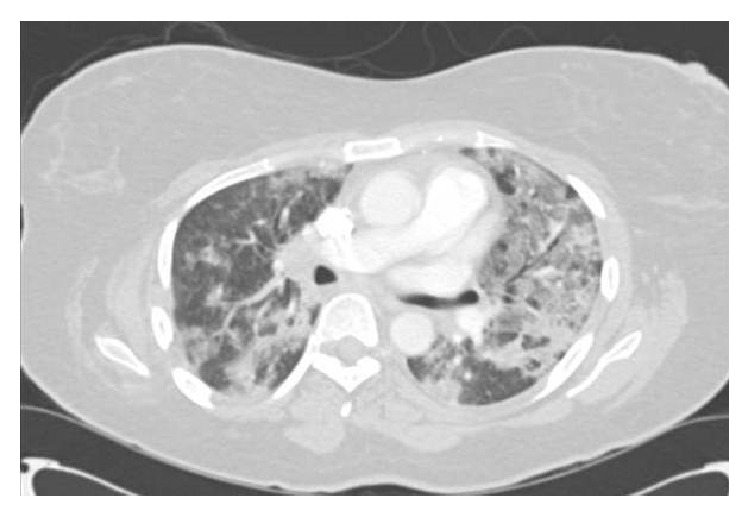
Showing computed tomography. There is moderate right hilar and mediastinal lymphadenopathy measuring up to 1.6 cm in short axis in the right hilum. The heart is moderately enlarged in size. There is a mosaic pattern of fairly dense opacity seen diffusely bilaterally.
